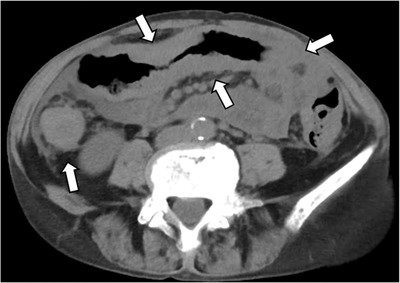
The computed tomography scan showed extensive wall thickening of the transverse colon to the ascending colon.
